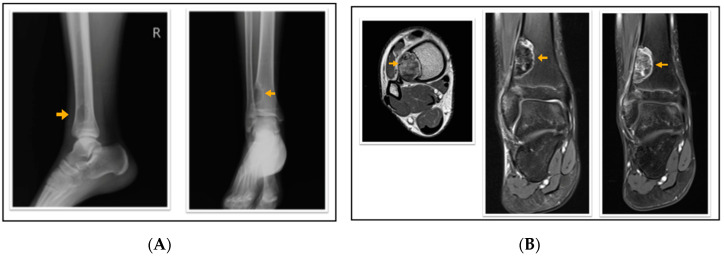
A 16-year-old patient with a fibrous cortical defect in the ankle. (A) Lateral and anteroposterior direct X-ray of a 16-year-old patient shows a lytic lesion with a sclerotic rim in the distal medial metaphysis of the tibia consistent with FCD. (B) Axial T1 weighted, coronal proton density, and coronal contrast-enhanced fat-saturated T1 weighted magnetic resonance images show sclerotic, non-contrast enhancing areas within the lesion. This lesion was classified as stage C according to Ritschl’s classification. Clinically, IPAQ (International Physical Activity Questionnaire):Inactive, Screen Time: 4 h, 21-Numbered Circle VAS (21-VAS): 3, VAS (Visual analogue scale): 3, 21-Numbered Circle Activity Scale (21-NCAS): 5.
